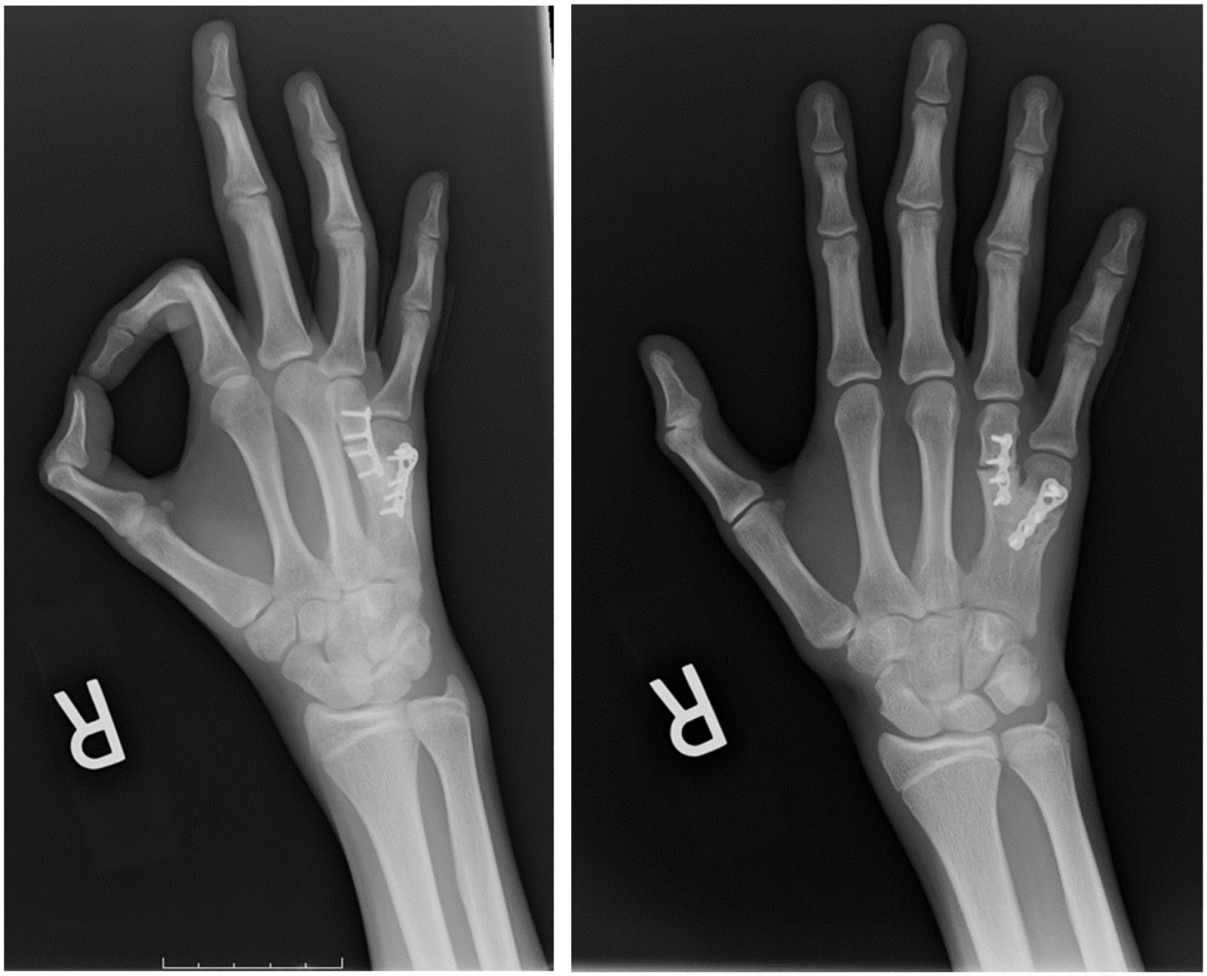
Postoperative radiographs of the patient’s right hand taken at 4 months after surgery.
